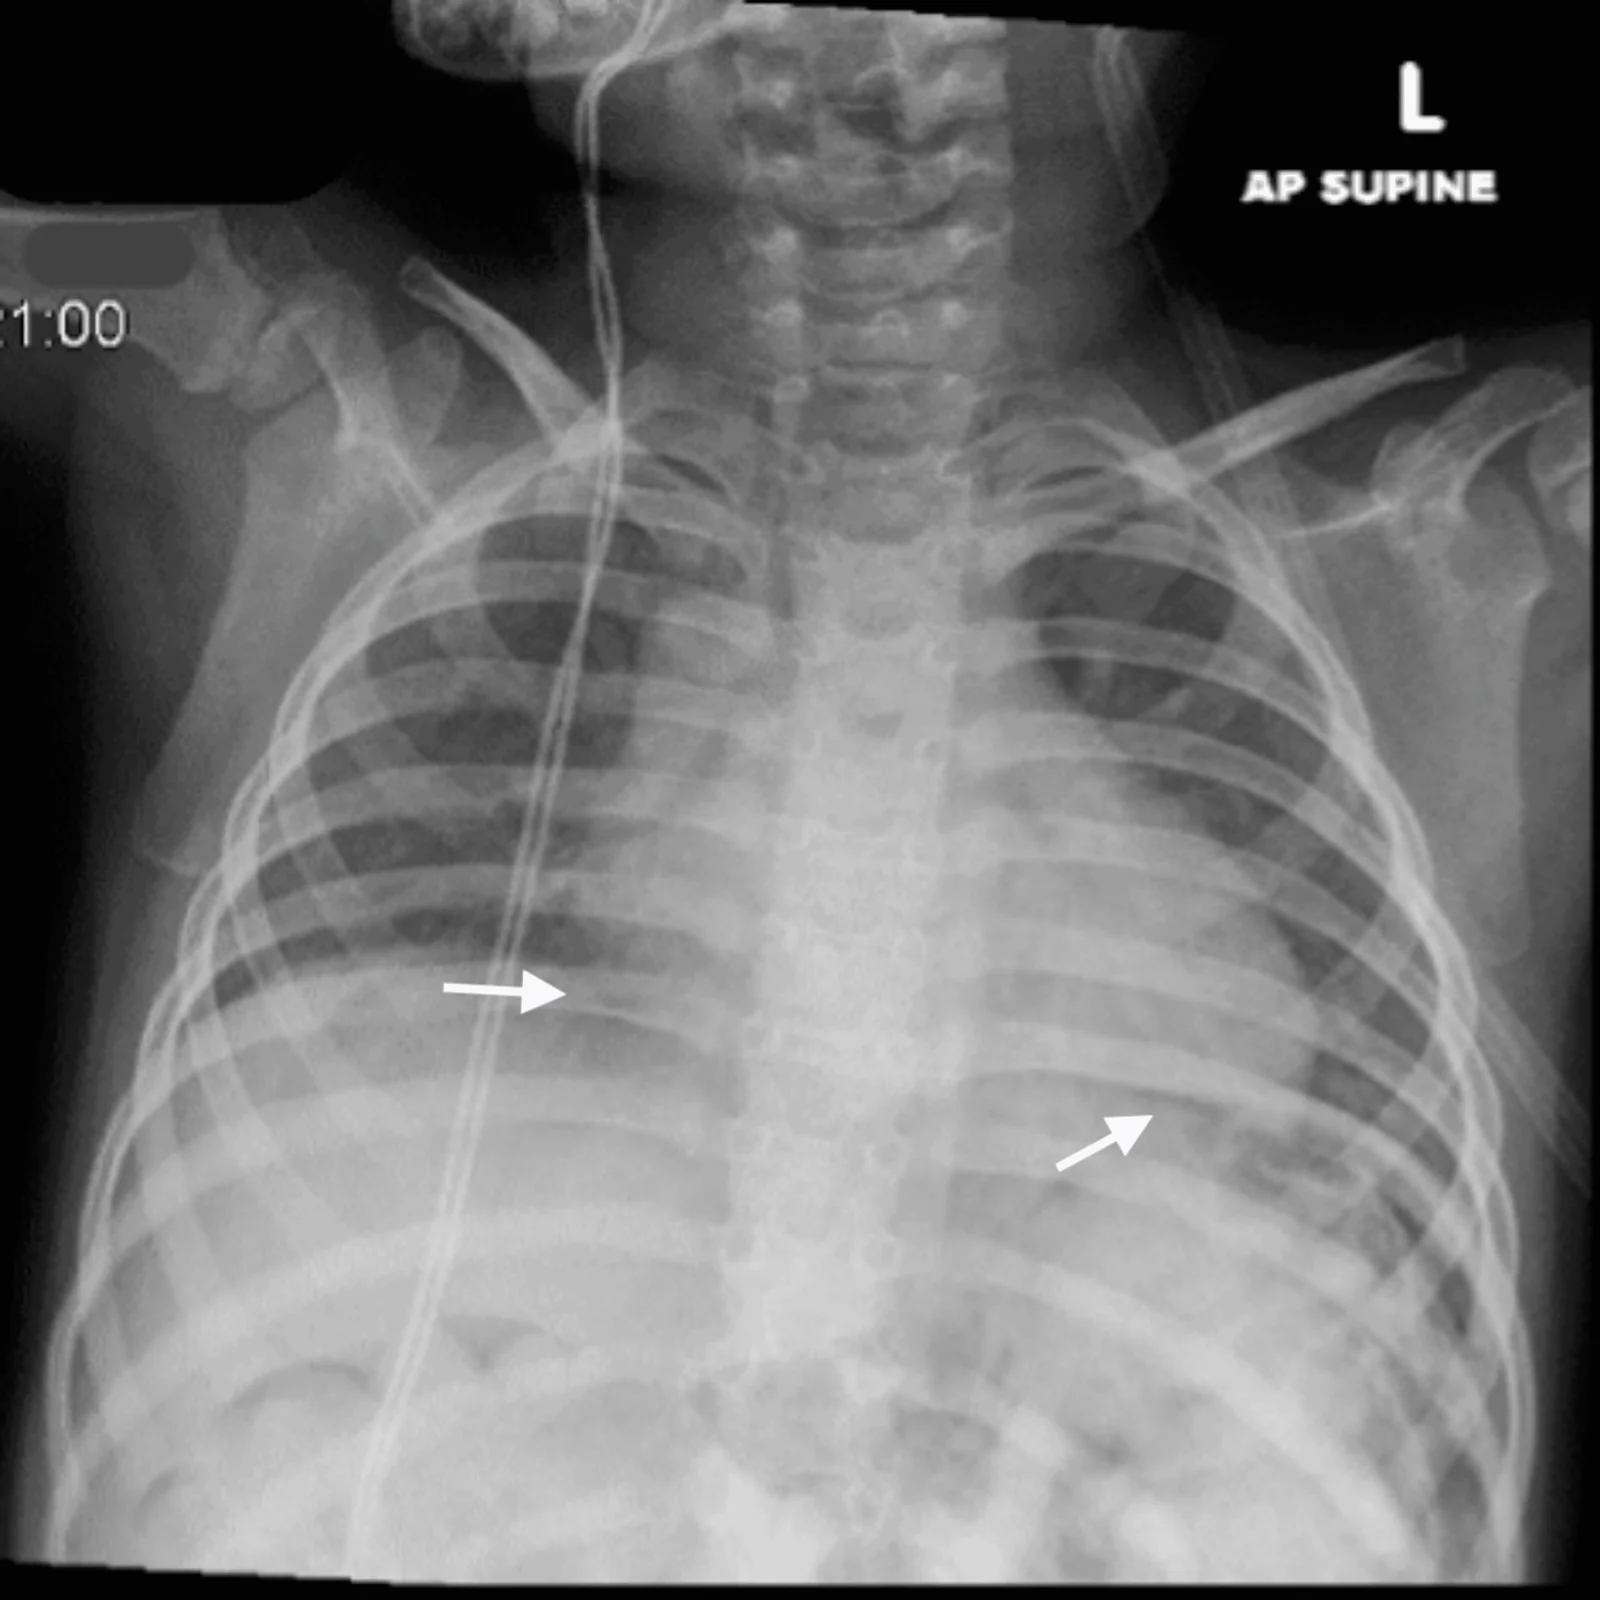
X-ray chest Day 2 post admission: free gas under both hemidiaphragms (white arrows), low lung volumes, no pleural effusion or pneumothorax
